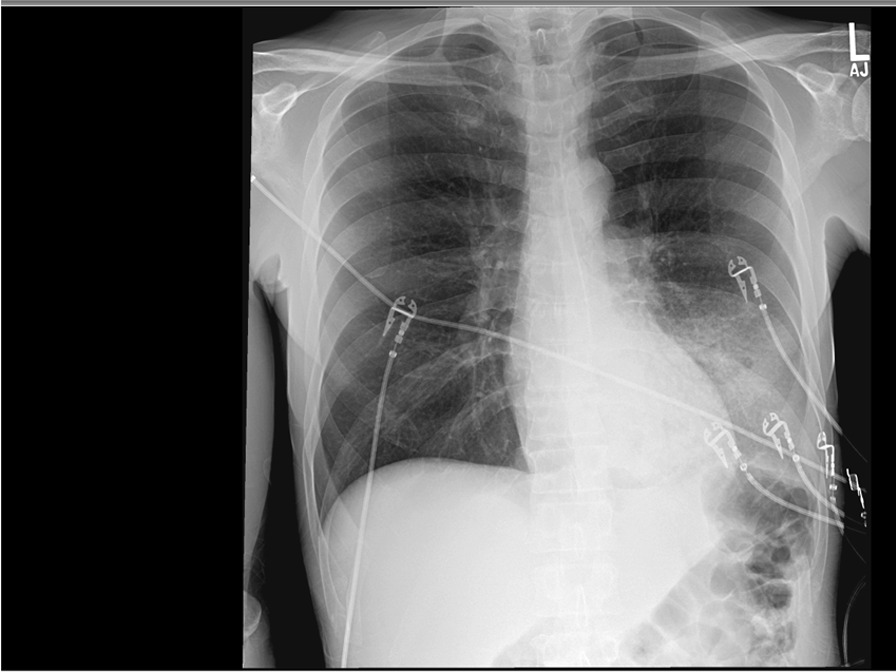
Chest X-ray on admission showing dense left lower lobe consolidation
